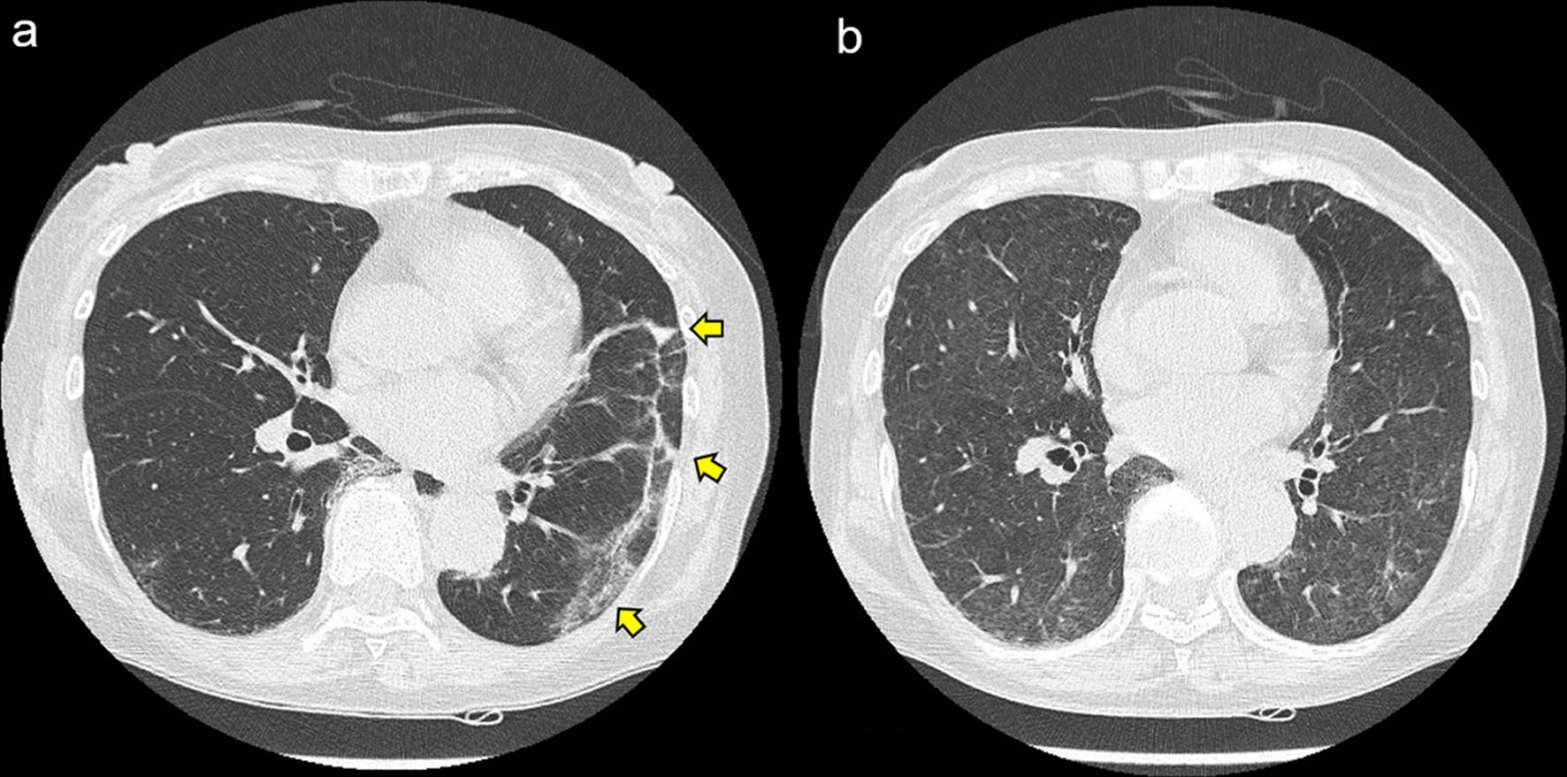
Chest computed tomography scan showing a ground-glass appearance with consolidation (yellow arrows) in the subpleural areas of the left lobe at the onset of COVID-19 (a), and the findings have disappeared in a month (b)
